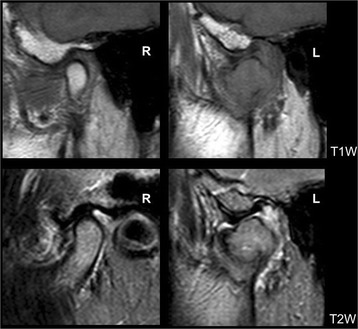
Magnetic resonance image. The upper images were T1-weighted and the lower images were T2-weighted. On the right side, the shape of the condyle was normal. An unstructured mass was observed in the left condylar region. A normal-shaped articular disc was seen on the destructed condyle
